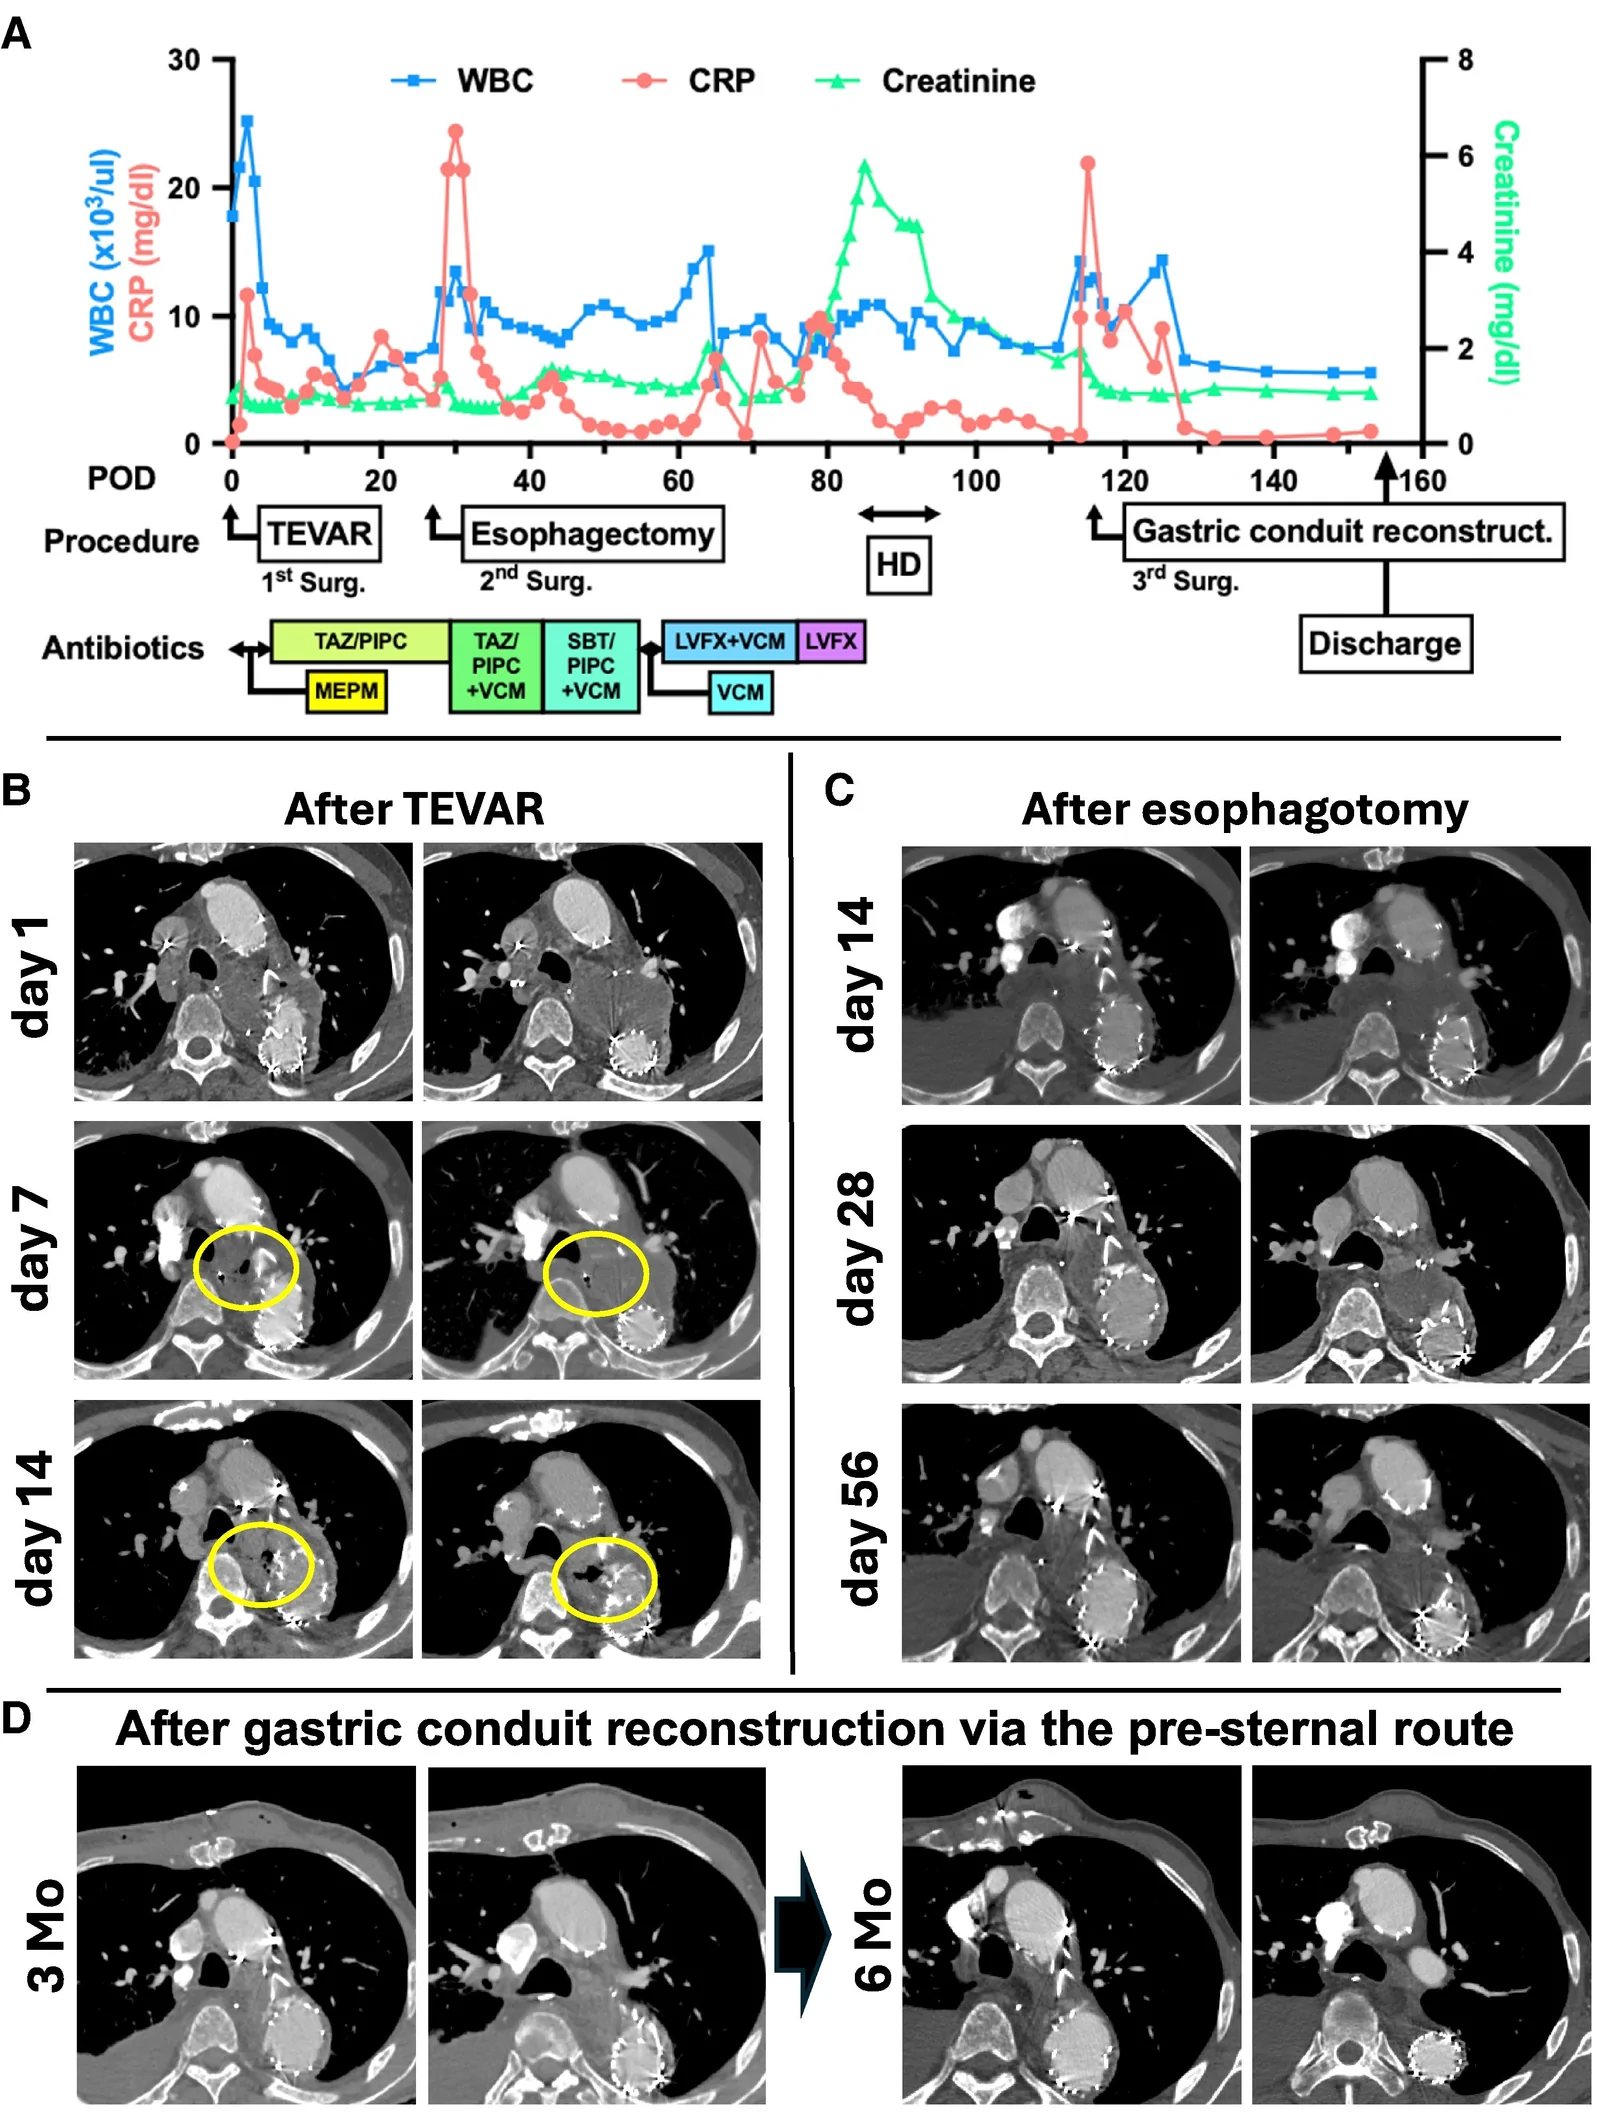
Computed tomography and aortography images. (*A*) The clinical course is shown. After thoracic endovascular aortic repair, antibiotic therapy was initiated. Despite the need for modification of antibiotics because of drug eruption and worsening renal function, inflammatory markers gradually improved after oesophagectomy. Antibiotics were discontinued 2 months after the second-stage surgery (∼3 months after thoracic endovascular aortic repair), and gastric conduit reconstruction via the pre-sternal route was performed at 4 months. The patient was discharged without evidence of recurrence. (*B*) A comparative computed tomography scan conducted after thoracic endovascular aortic repair demonstrates a progressive increase in the presence of free air within the mediastinum surrounding the oesophagus and pseudoaneurysm cavity (yellow circles). (*C*) After oesophagectomy, computed tomography confirms resolution of mediastinal free air. (*D*) Following gastric conduit reconstruction via the pre-sternal route, no recurrence is observable on computed tomography. HD, haemodialysis; CT, computed tomography; TEVAR, thoracic endovascular aortic repair; TAZ/PICC, tazobactam/piperacillin; MEPM, meropenem; VCM, vancomycin; SBT/PIPC, sulbactam/piperacillin; LVFX, levofloxacin.
